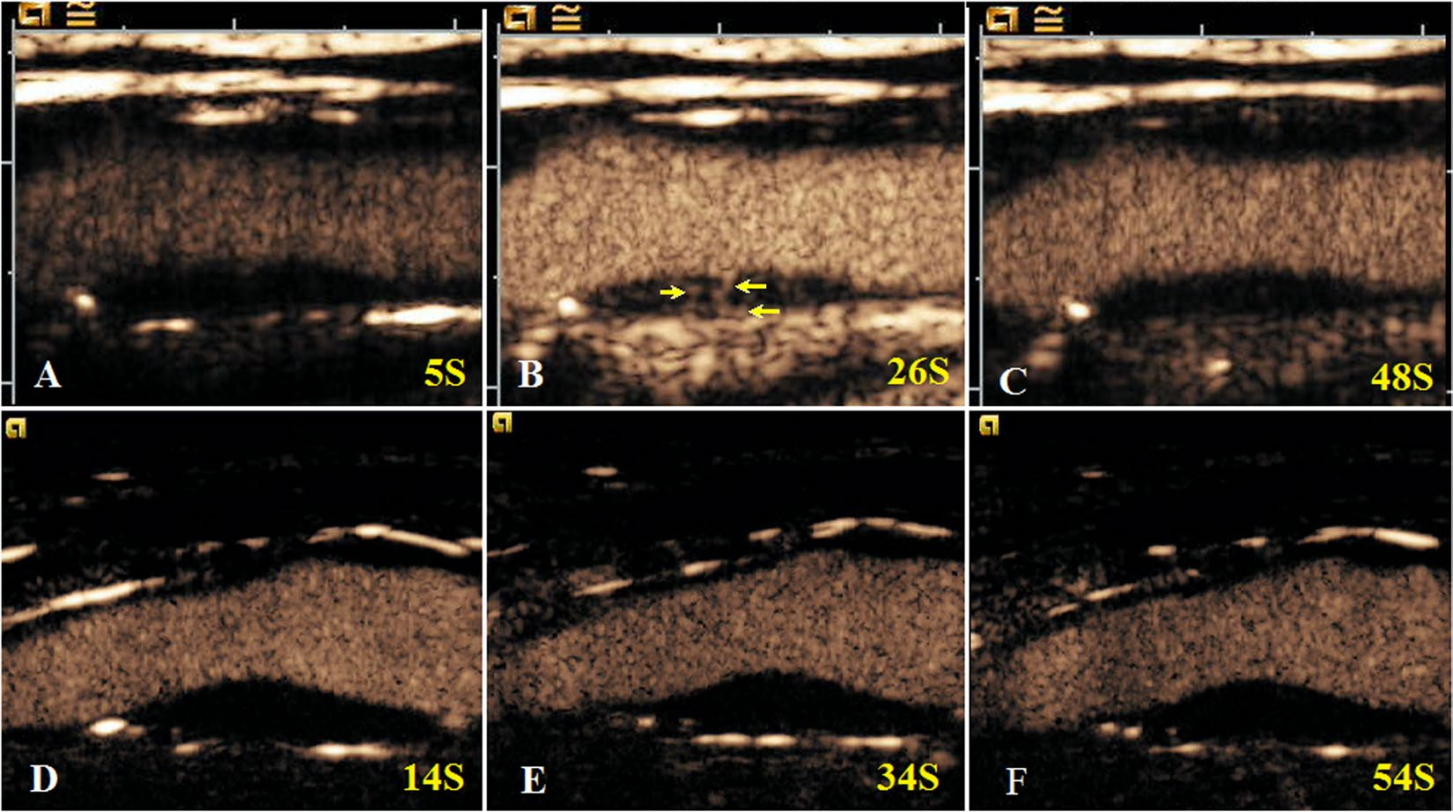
Contrast-enhanced ultrasound (CEUS) of intraplaque neovascularization (IPN) in carotid arteries of a patient with both acute ischemic stroke (A–C) and a patient without acute ischemic stroke (D–F). IPNs were observed in the plaque ROI (yellow arrows).
